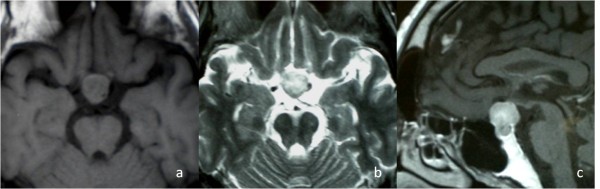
Initial magnetic resonance imaging. A) T1-weighted magnetic resonance image showing an isointense pituitary mass. B) T2-weighted magnetic resonance image showing a hyperintense mass.
